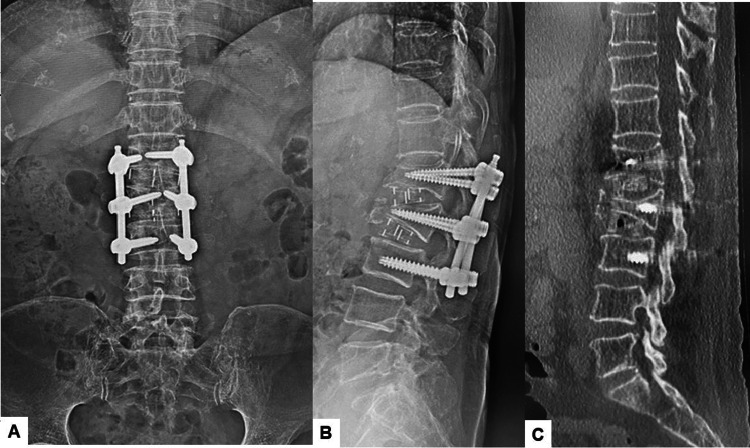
Postoperative radiograms and CT (A) Anteroposterior lumbar radiogram. (B) Lateral lumbar radiogram. (C) Mid-sagittal reconstruction CT.
